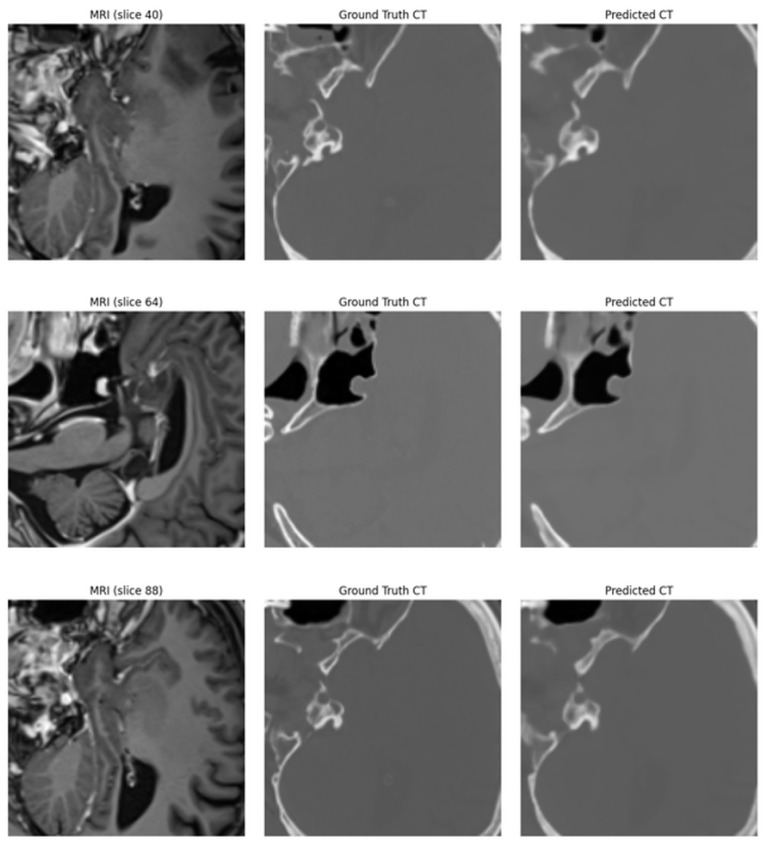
The visual comparison between the brain MRI image inputs (first column), actual CT images (second column), and predicted CT images (third column) across different brain slices (at levels 40, 64, and 88). The optimized 3D U-Net model demonstrates strong reconstruction capabilities, especially in high-density structures such as the skull and facial bones. In complex anatomical regions, some slight differences in soft tissue can be observed, but overall structural integrity remains well preserved. These results highlight the clinical potential of the model for generating reliable CT images from MRI data, particularly when CT imaging is unavailable.
